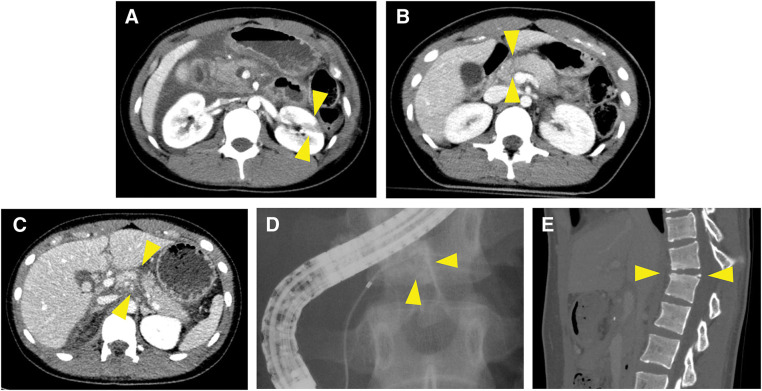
Images before the surgical intervention in Case 2. (A) Contrast-enhanced CT scan after admission to the previous hospital. The arrowheads mark the kidney injury (AAST-OIS grade IIIb). (B) Contrast-enhanced CT scan after admission to the previous hospital. The arrowheads mark a slight abnormality of the pancreatic injury. (C) Contrast-enhanced CT scan after admission to our hospital (3 days after injury). The arrowheads mark the location of the pancreatic injury, which is more pronounced compared to (A). (D) ERP revealed the leakage of contrast agents from the MPD. (E) Sagittal CT scan of dislocation fracture of the 11th and 12th thoracic vertebrae. AAST, American Association for the Surgery of Trauma; CT, computed tomography; ERP, endoscopic retrograde pancreatography; MPD, main pancreatic duct
